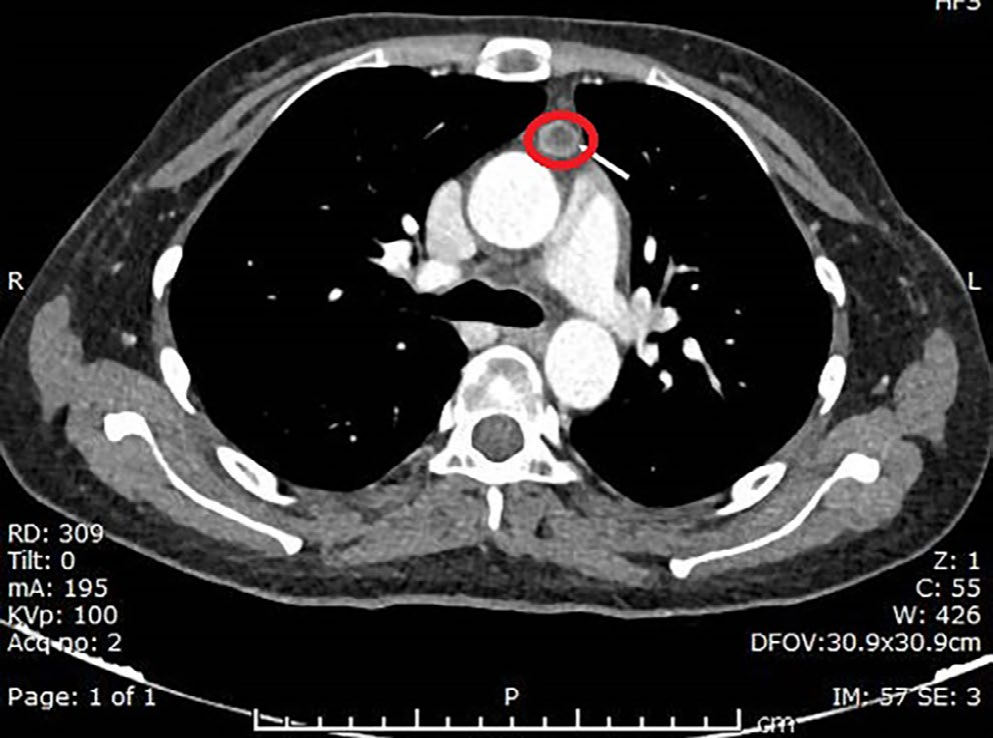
Axial contrast‐enhanced CT study at the level of midthoracic regions showing a small peripherally enhancing solid abnormality within the thymus circle
